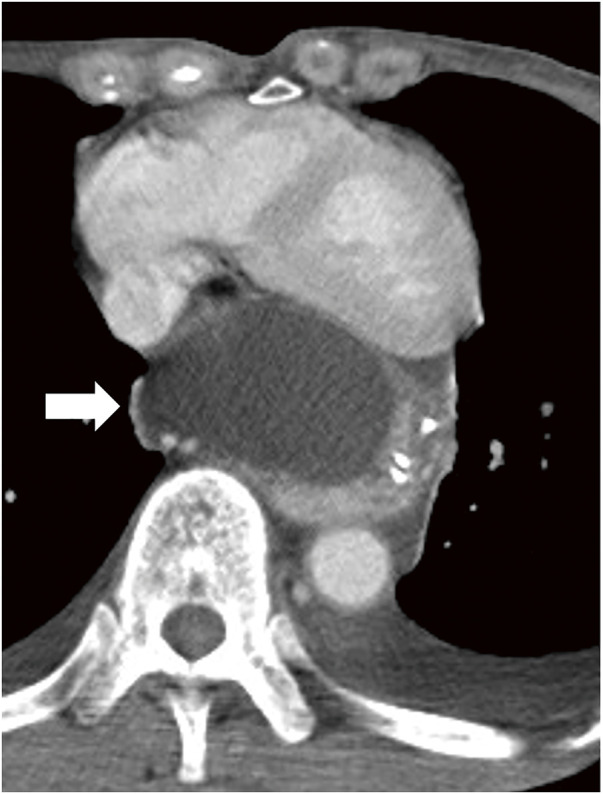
Mediastinal CT image on POD12. The low-attenuation area (white arrow) in the mediastinum, indicative of lymphatic leakage, compresses the pericardium.
